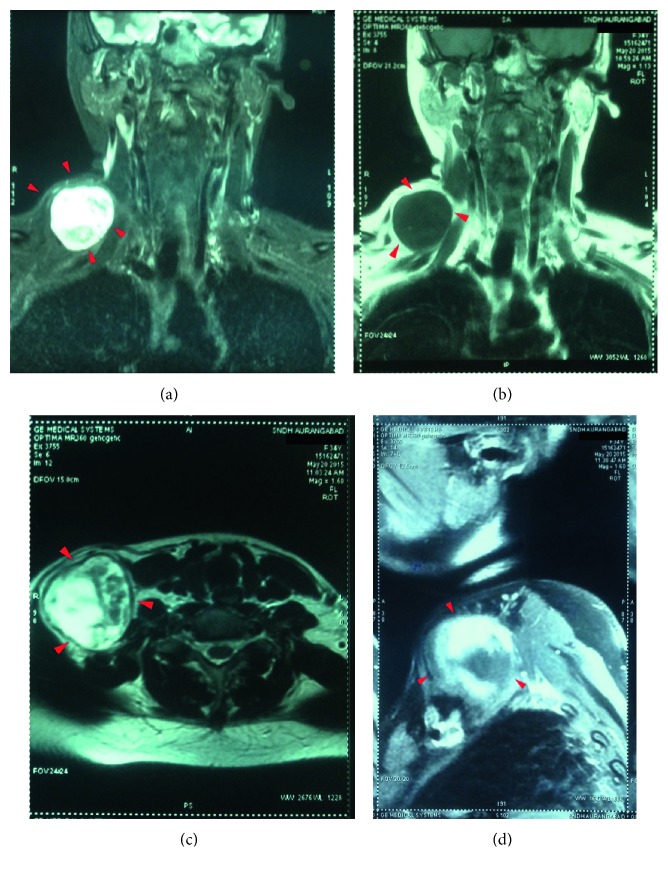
(a) T2-weighted coronal cut. (b) T1-weighted coronal cut. (c) T2-weighted axial cut. (d) Postcontrast T1 sagittal cut.
